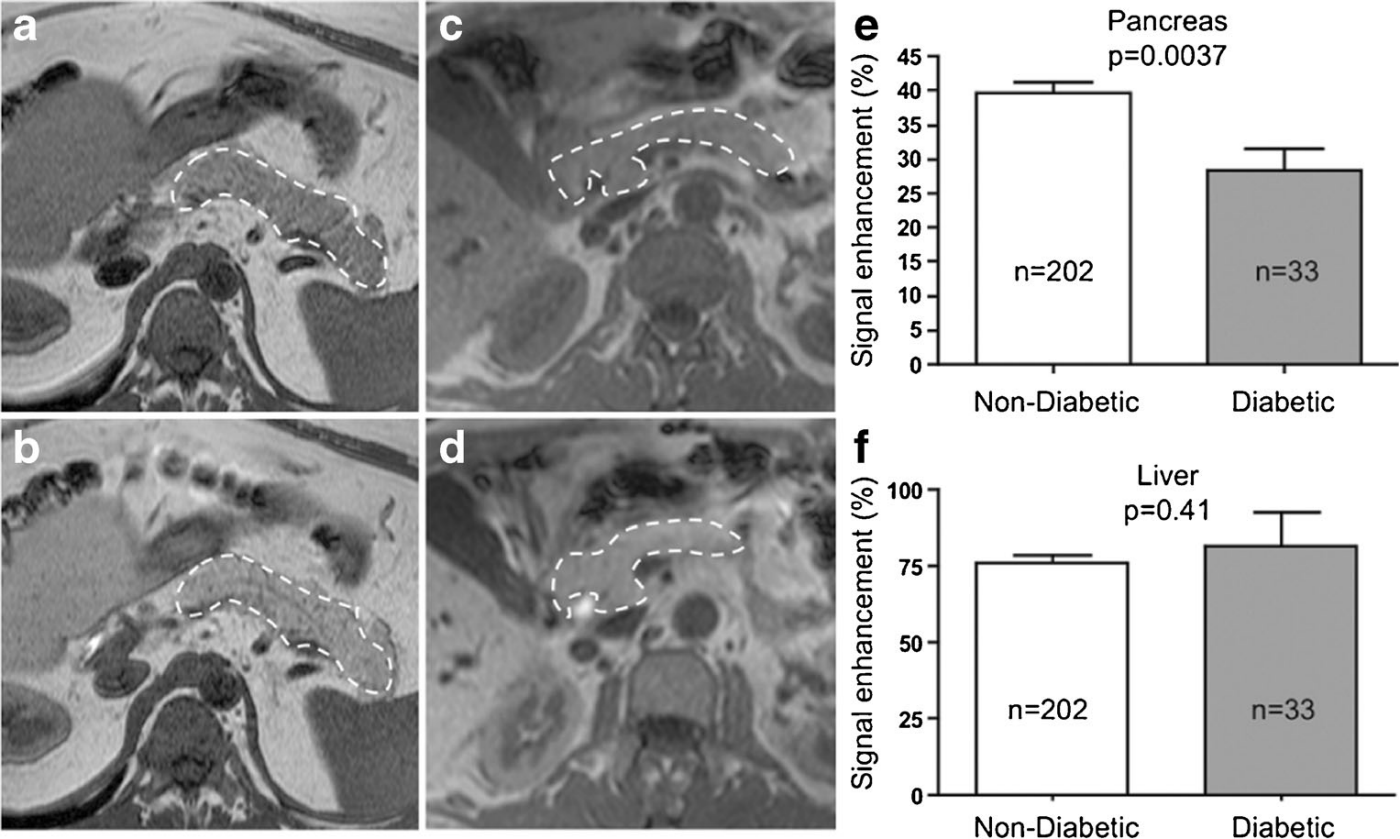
Mn^2+^-enhanced MRI of the pancreas (*dashed lines*). a, c Diabetic patient. b, d Normoglycemic patient. e Signal enchantment was significantly higher in normoglycemic patients [47]
